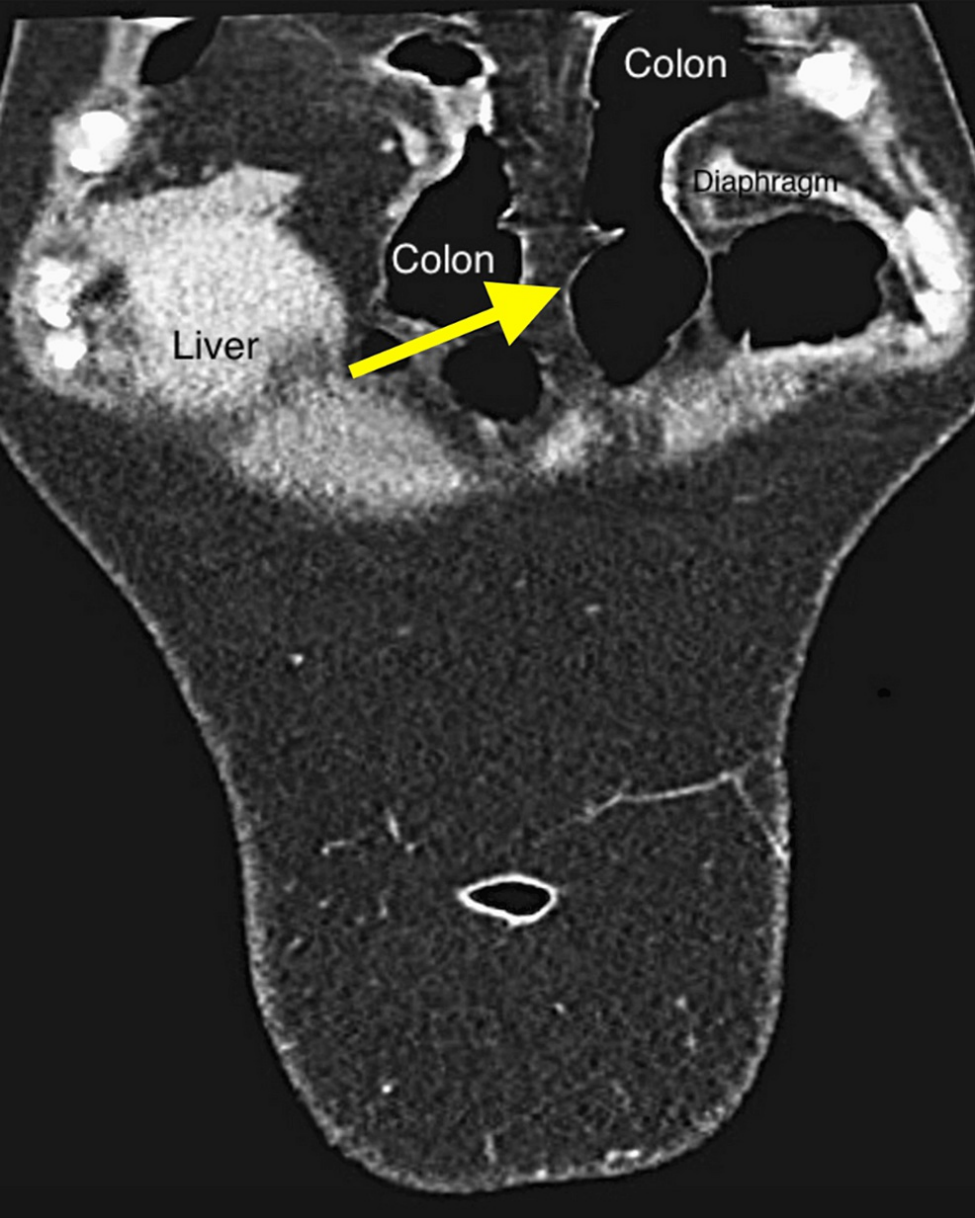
Frontal view of abdominal and thoracic CT scan showing an anterior diaphragmatic hernia containing transverse colon and greater omentum (yellow arrow)
